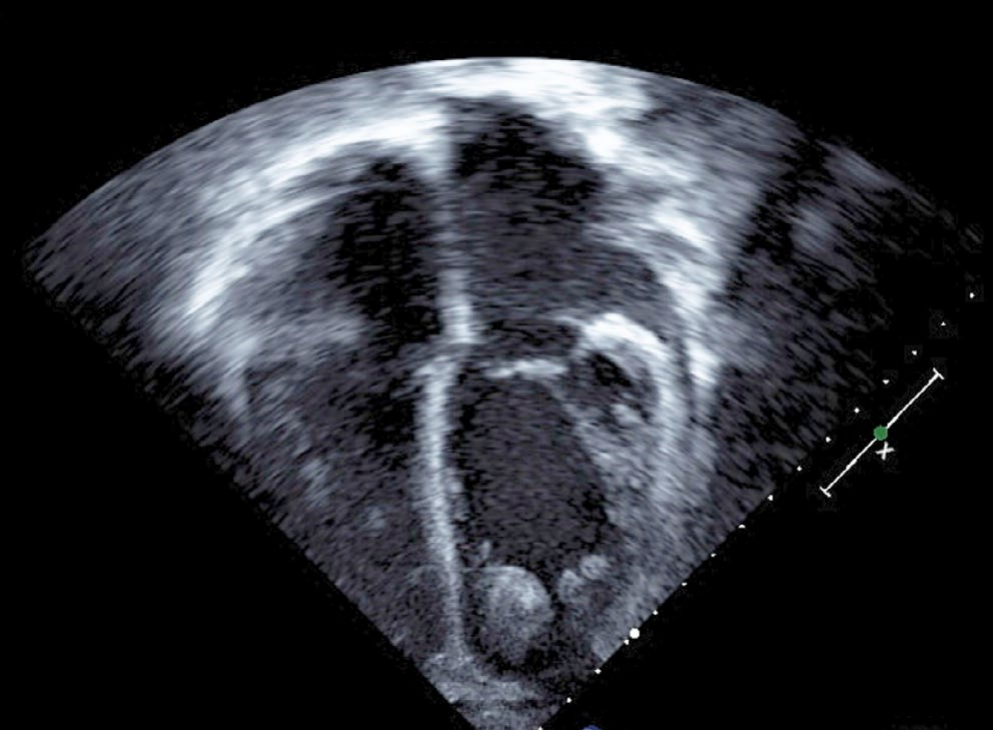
Echocardiography of Case 1 showing Multiple clots seen in left ventricular apex
